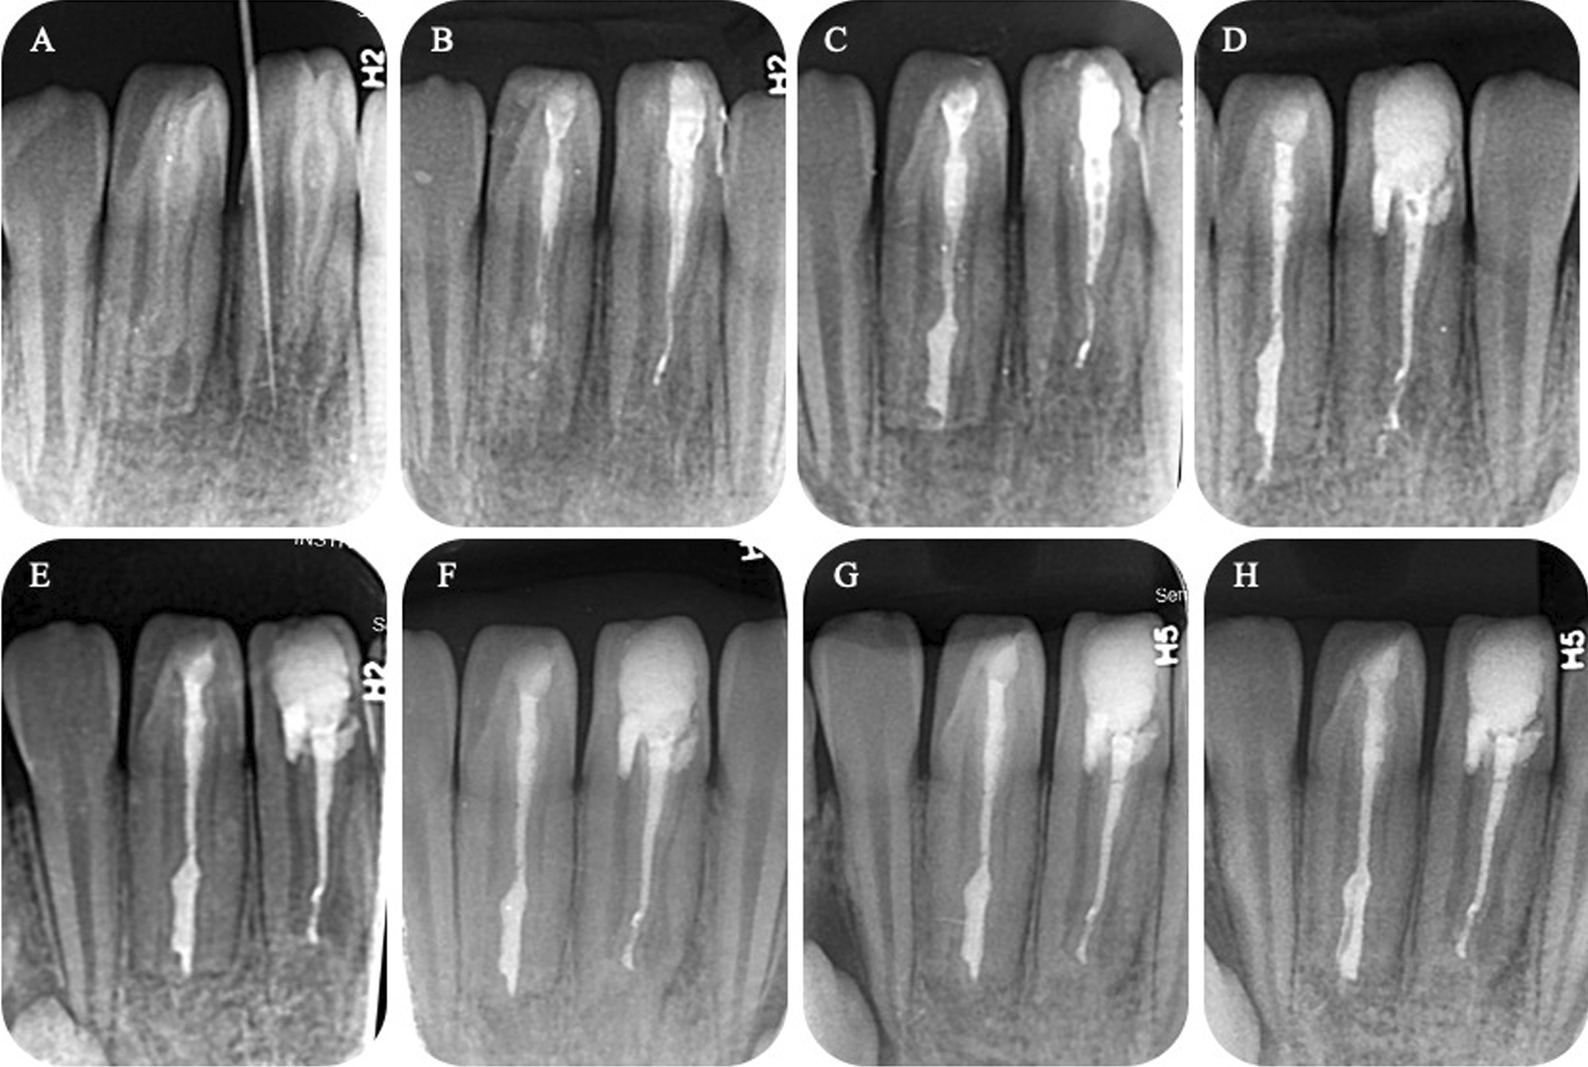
Treatment and follow-ups periapical radiographs. A preoperative, B postoperative after the first vitapex filling, C postoperative after the second vitapex filling, D postoperative after iRoot BP plus filling of the main root canal and iRoot SP and warm gutta-percha obturation of the invaginated root canal (#31), and at E 5-month, F 1-year, and G 1.5-year recall. The radiographic image indicates apex closure of the main root canal and root development of the tooth. H Postoperative after iRoot SP and warm gutta-percha obturation of the invaginated root canal (#41)
